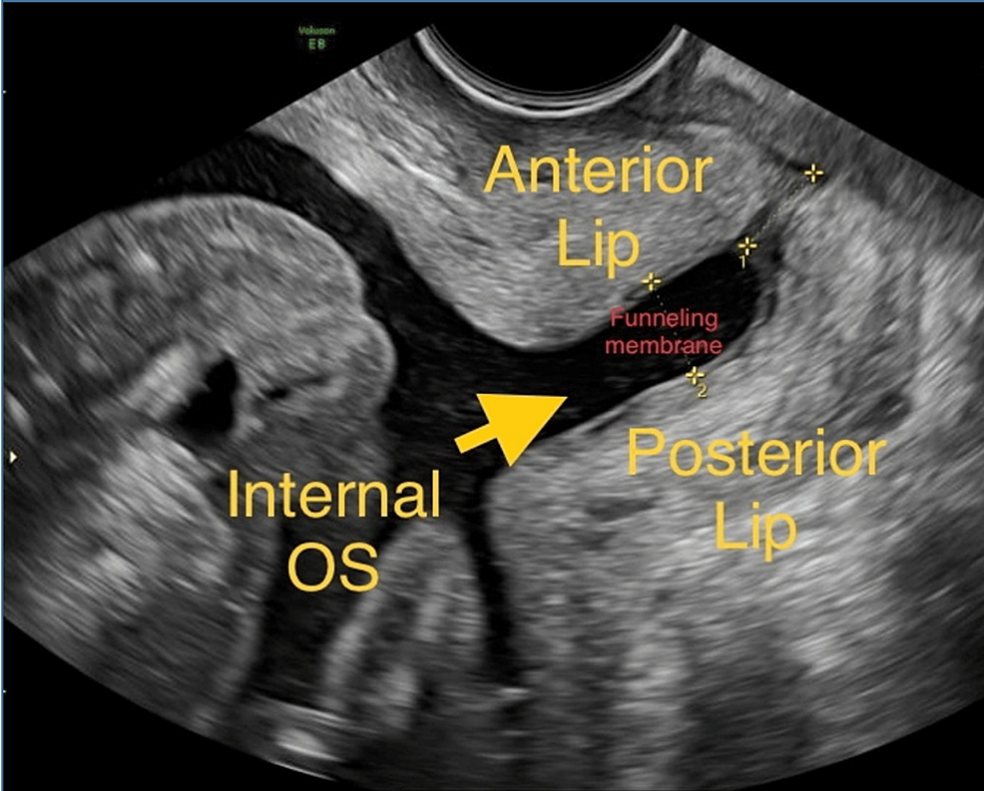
Ultrasound image showing short cervix with funneling of membranes.
